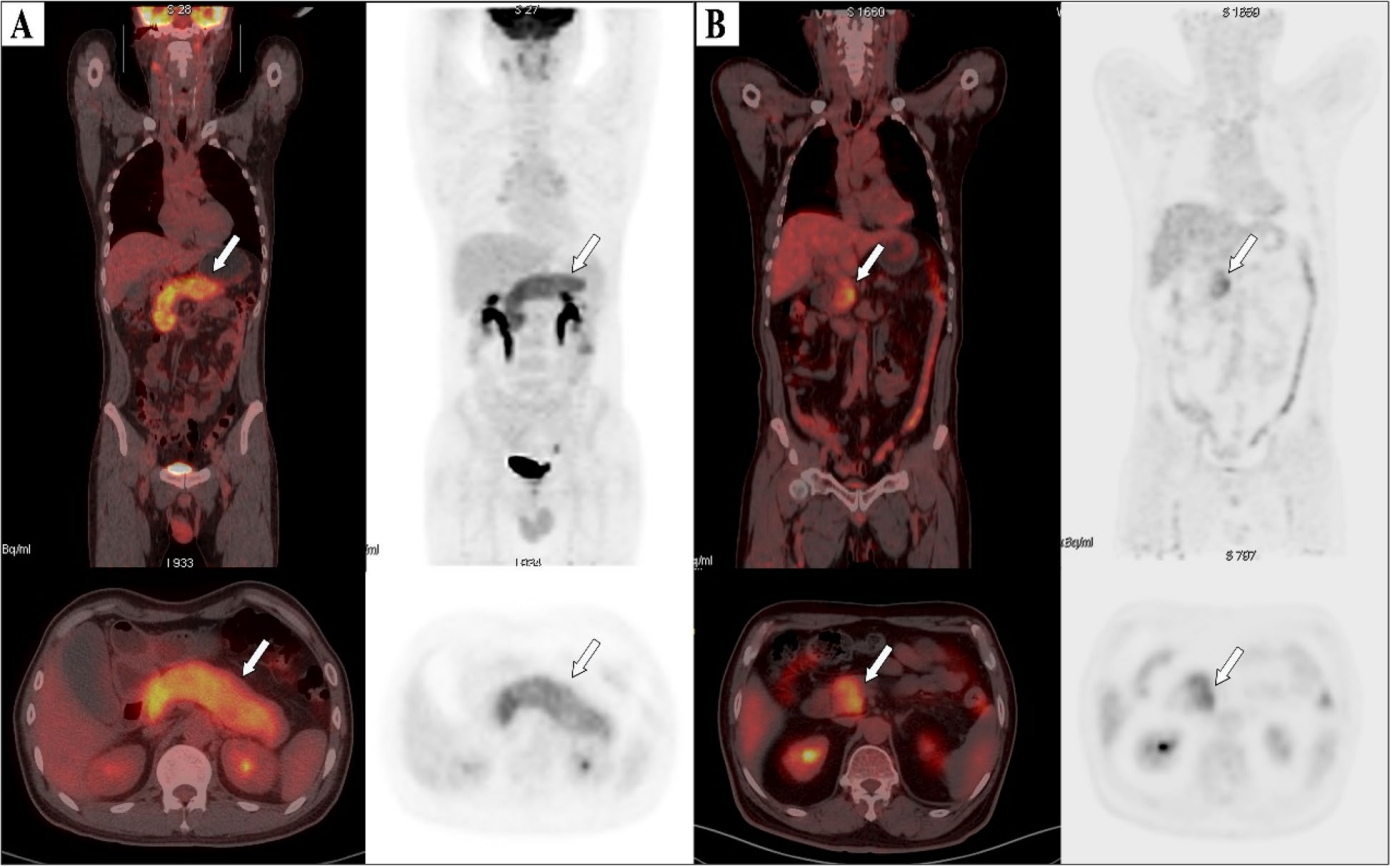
^18^F-FDG PET-CT images of patients. A Image of a 61-year-old male patient with type 1 AIP, demonstrating diffuse enlargement of the pancreas. (arrows). The serum IgG4 level was 2260.0 mg/dL, and the TLG_2.5_ was 413.8 g/ml×cm^3^. According to the Nomogram, his relapse probability is more than 95%. The patient discontinued steroid use during the maintenance phase, and 7 months later, he relapsed, with imaging showing diffuse re-enlargement of the pancreas. B Image of a 60-year-old male patient with type 1 AIP, showing focal enlargement in the head of the pancreas (arrows). The serum IgG4 level was 980 mg/dL, and the TLG_2.5_ was 92.7.0 g/ml×cm^3^. According to the Nomogram, the patient’s relapse probability is less than 50%. After symptom relief, the patient did not receive maintenance treatment, and has been followed up for 4 years without relapse
